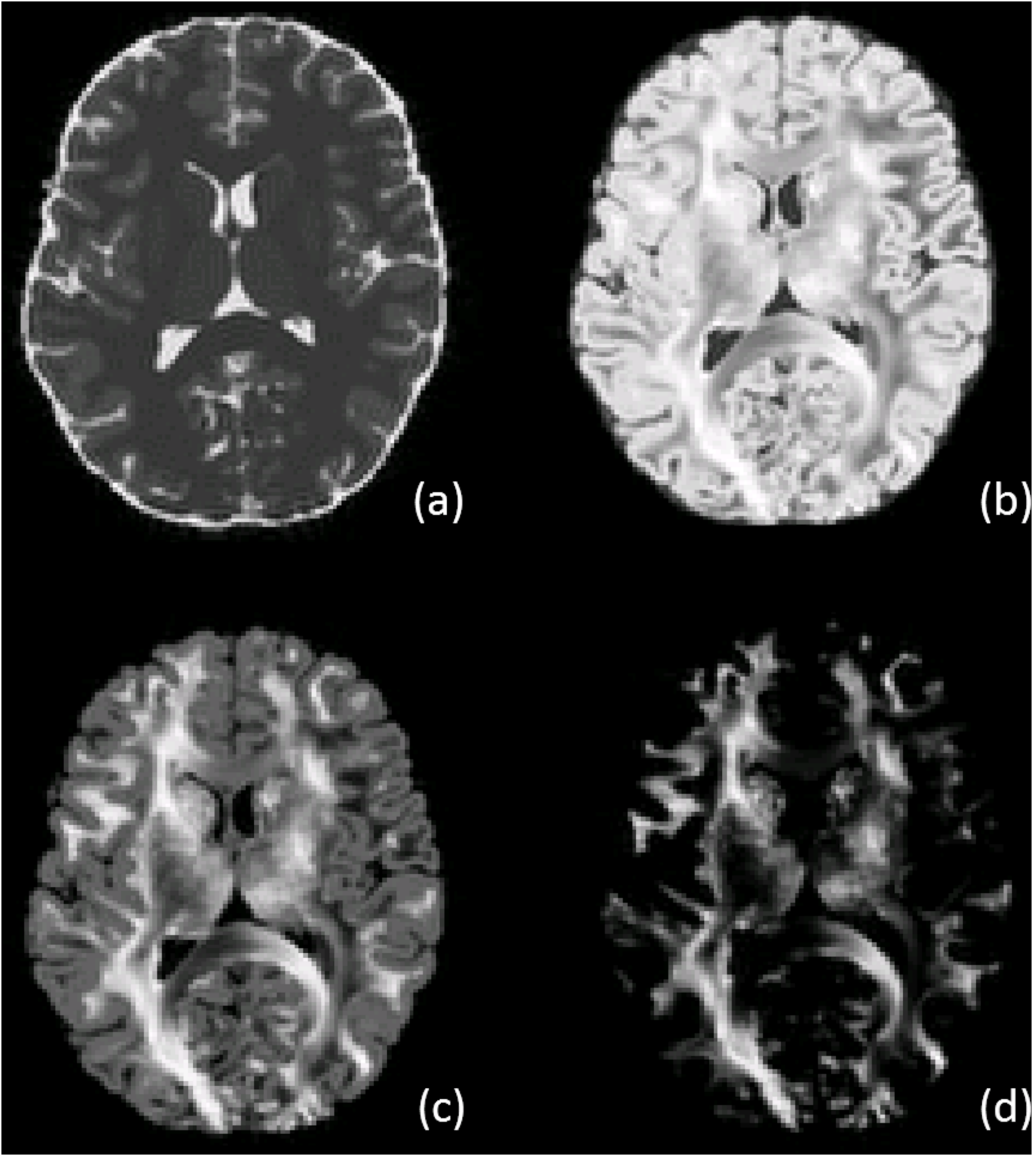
D-BRAIN. Anatomical MR image (a) and corresponding diffusion-weighted images for D-BRAIN data. B-values of 1000 s mm^−2^ (b), 2500 s mm^−2^ (c) and 10000 s mm^−2^ (d). For each picture, the intensity values have been optimized for visual purposes.
